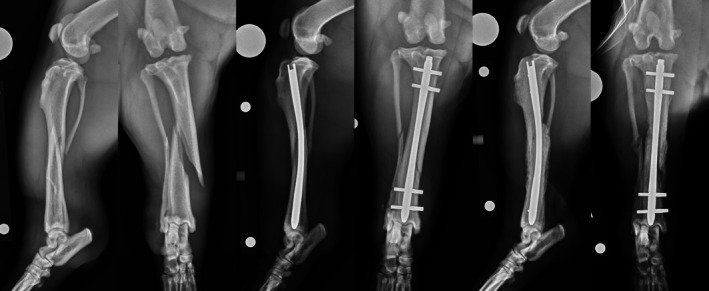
Nail bending. Preoperative, immediate and 6‐week postoperative radiographs of a 1‐year‐old Pitbull with a Gustilo‐Anderson type I open long oblique fracture of the mid tibial diaphysis. The fracture was stabilized using a 6 × 122 mm I‐Loc AS‐ILN in 2:2 configuration bent to an angle of 168° to follow tibial recurvatum.
